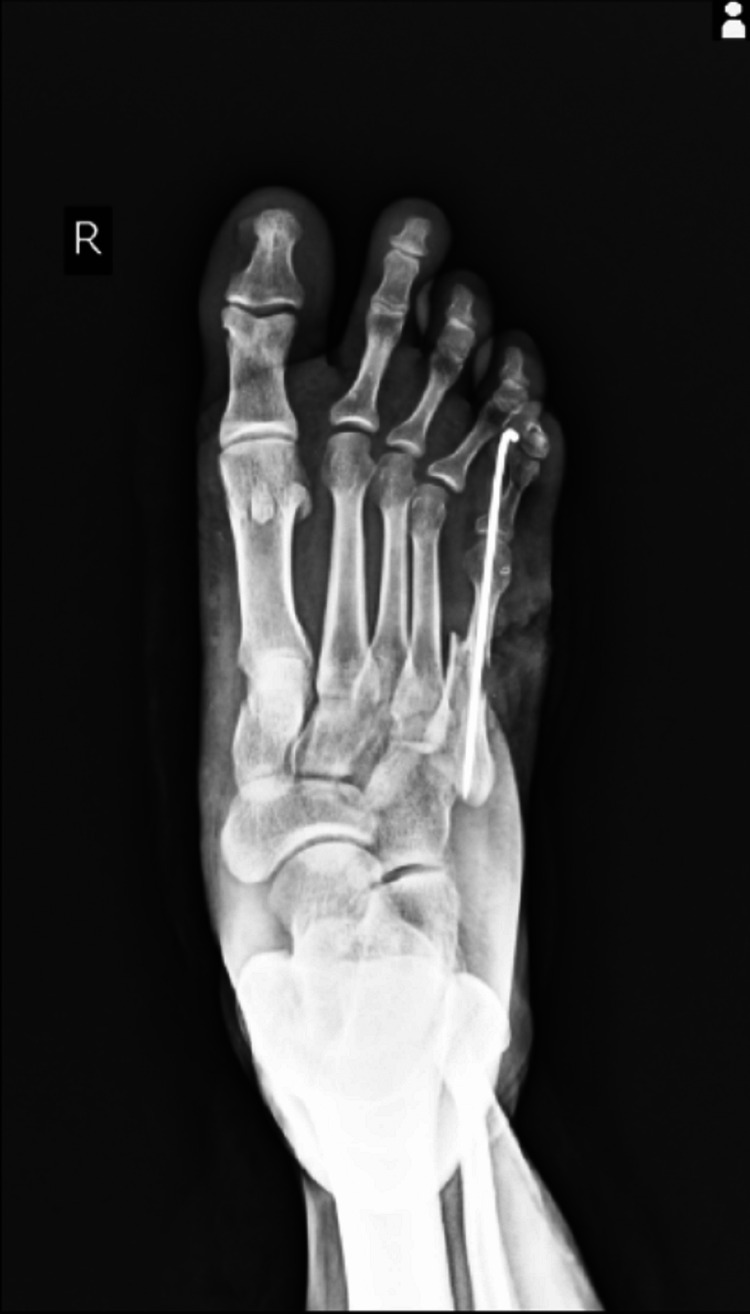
X-ray showing fifth metatarsal fracture managed with K-wire fixation - anteroposterior view
